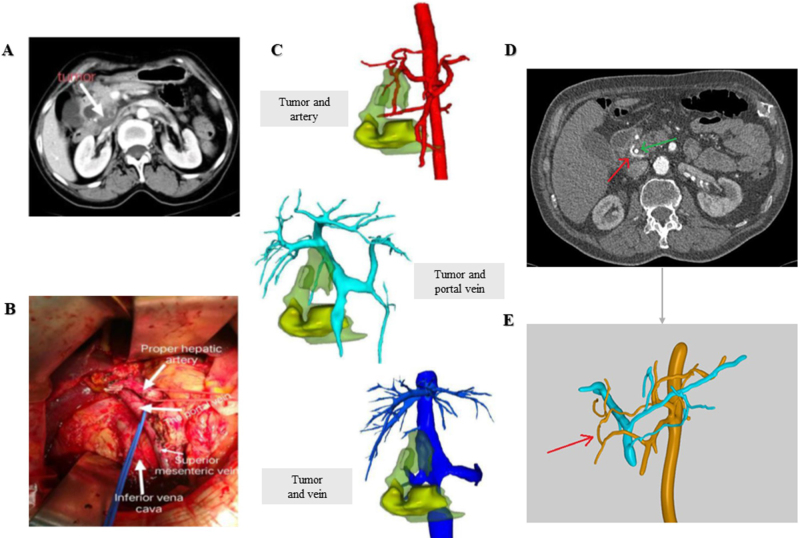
(A) CT scan of the patient); (B) intraoperative image after tumoral resection, with intact SMA, PV, superior mesenteric artery, and IVC; (C) Tumoral relationship with arterial vessels, veins, and portal vein. Images A, B, and C extracted from^[11]^; (D) CT scan showing Bühler anastomosis. Red arrow: vascular variant; green arrow: stent in the hepatic duct; (E) digital 3D model showing the Bühler anastomosis. Images D and E extracted from^[28]^.
